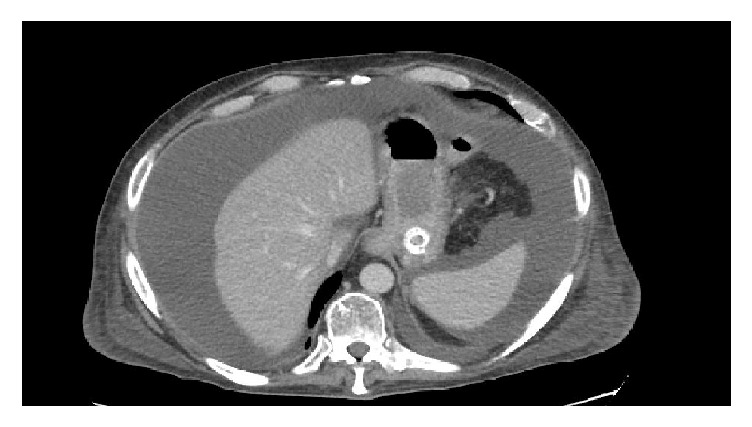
Patient 1, CT scan abdomen showing resolution of perigastric abscess.
